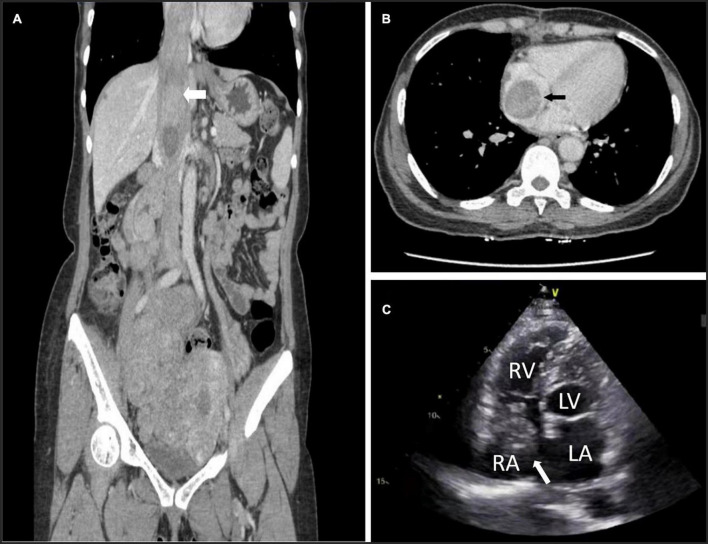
Preoperative computed tomography venography (CTV) and echocardiography can detect tumors. (A) Image of patient 5 showing intracardiac leiomyomatosis (ICL) extending through the inferior vena cava (IVC) to the right atrium (RA) (white arrow). (B) Image of patient 3 showing a tumor within the RA (black arrow). (C) Echocardiogram of patient 10 shows a solid intracardiac head. LA, left atrium (white arrow); LV, left ventricle; RV, right ventricle.
